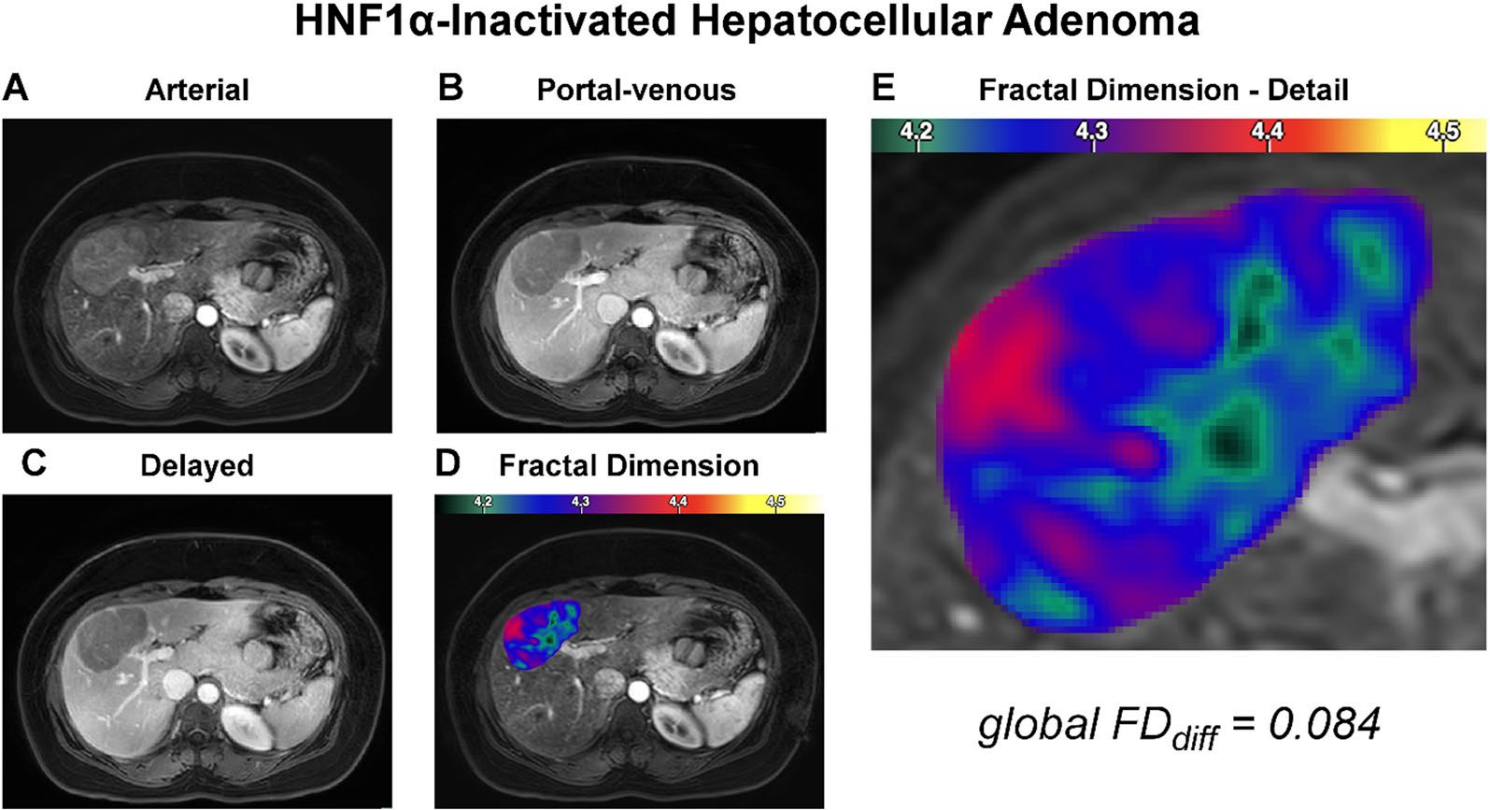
Representative case of hepatocyte nuclear factor (HNF)-1α-inactivated hepatocellular adenoma. Dynamic contrast-enhanced MR images of the arterial (A), portal venous (B), and delayed (C) phase are shown along with the fractal dimension (FD) map as overlay over the arterial phase (D) and a zoom on the lesion (E). The panel organization is the same throughout Figs. 2, 3, 4, and 5. The depicted patient was a 26-year-old female with obesity (BMI = 28 kg/m^2^) and no history of oral contraception. She was admitted for characterization of an incidentally found liver mass. Typical MRI features including arterial phase hyperenhancement, washout, and out-of-phase signal drop (not shown) suggested HNF1α-inactivated adenoma, which was subsequently confirmed by biopsy
